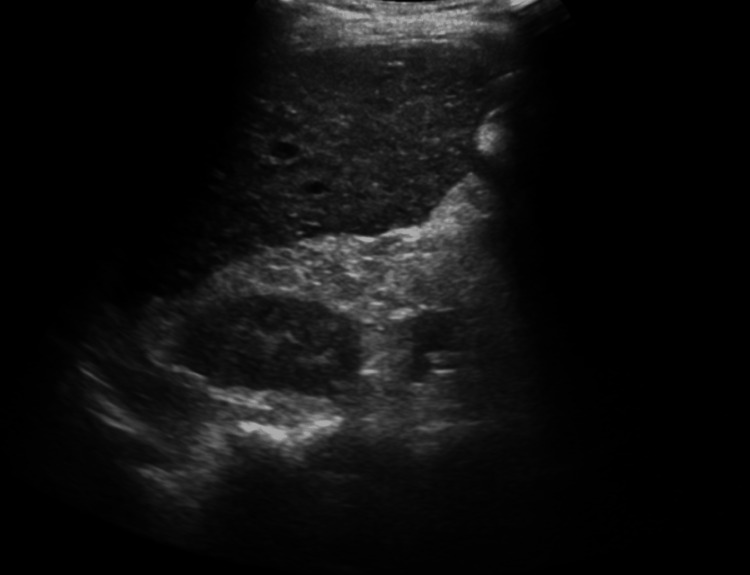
Abdominal ultrasound (sagittal view of the right lobe) showing heterogeneous hepatic parenchyma and a nodular liver contour consistent with cirrhosis.
